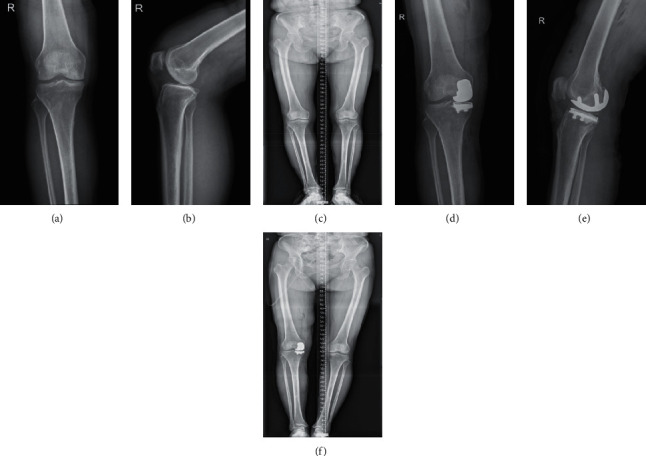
Patient, female, 53 years old. Pain and limited movement for more than 4 years, osteoarthritis of the right knee, unicondylar knee arthroplasty of the right knee. (a, b) Preoperative anteroposterior and lateral view of right knee joint. DR: the right knee joint was composed of complete bone, some bone edges showed hyperosteogeny, intercondylar protuberant hyperplasia, joint space existed, and there were no obvious abnormalities in surrounding soft tissues. Degeneration of the right knee. (c) Preoperative full-length X-ray of lower limbs showed narrowing of the medial joint space of the right knee joint and valgus deformity. (d, e) Anteroposterior and lateral radiographs of the right knee joint after partial replacement of the right knee joint: the replacement was in place and the joint space was called. (f) Postoperative full-length X-ray of lower limbs showed that the knee valgus deformity was corrected, the prosthesis was in a good position, and the force line of lower limbs was restored.
